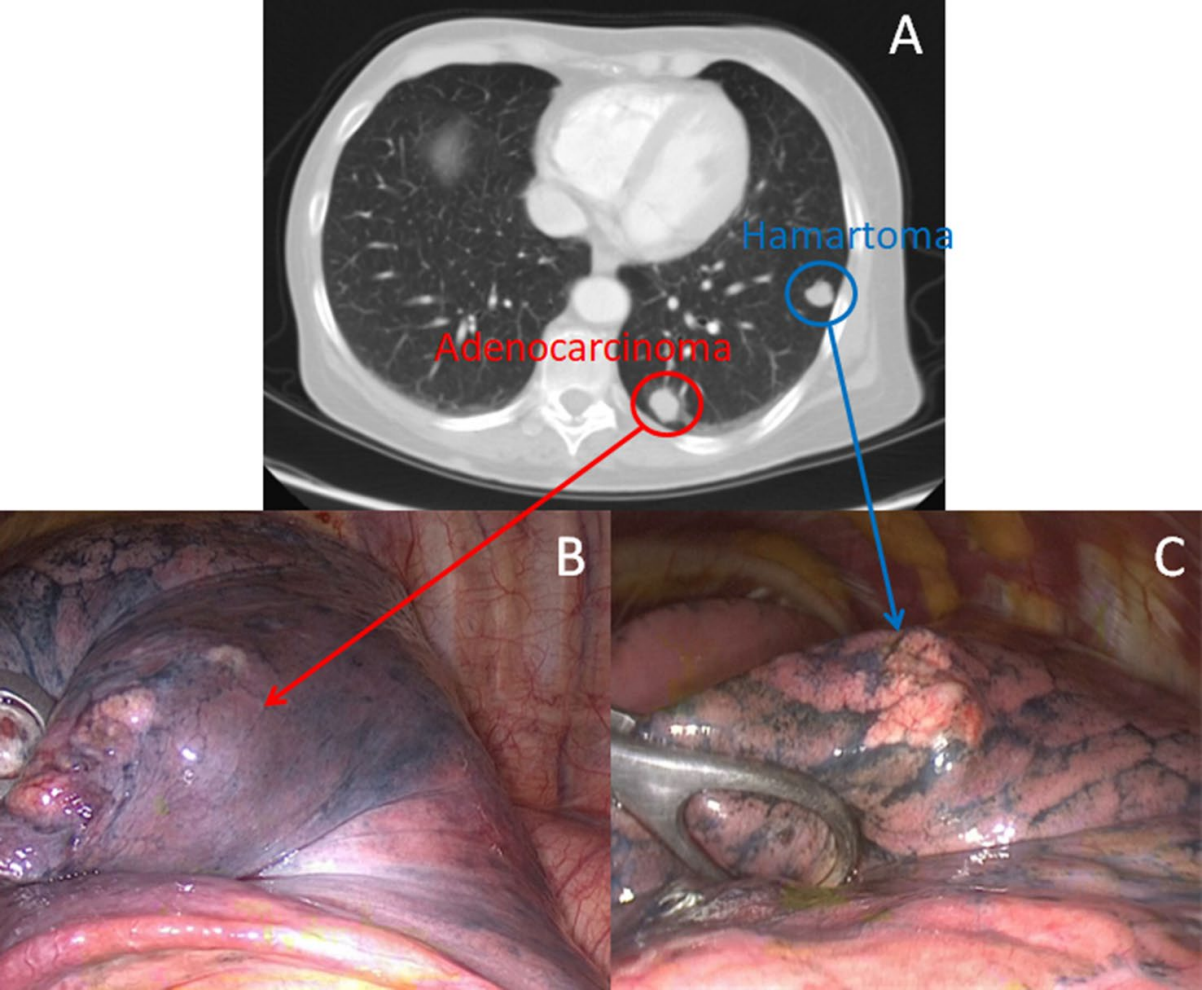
A 57-year-old woman had hamartoma and adenocarcinoma in the left lower lobe. She underwent thoracoscopy left lower lobectomy. Part A = CT scan; Part B and Part C = operative view of adenocarcinoma (B) and hamartoma (C)
